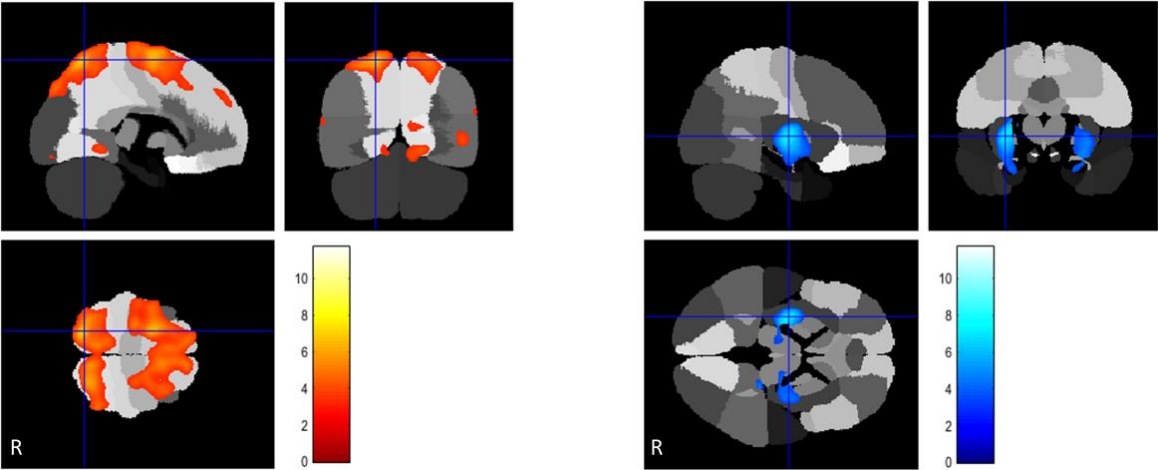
FDG uptake in CP-term cases (*n* = 45) compared to controls. *Left*: Regionally increased uptake in the superior anterior frontal and superior parietal lobules respectively, displayed in a yellow-scale with brighter tones indicating higher *t*-scores. *Right*: Regionally reduced uptake in the posterior putamina, globi pallidi and lateral parts of the thalami displayed in a blue-scale with brighter tones indicating higher *t*-scores.
